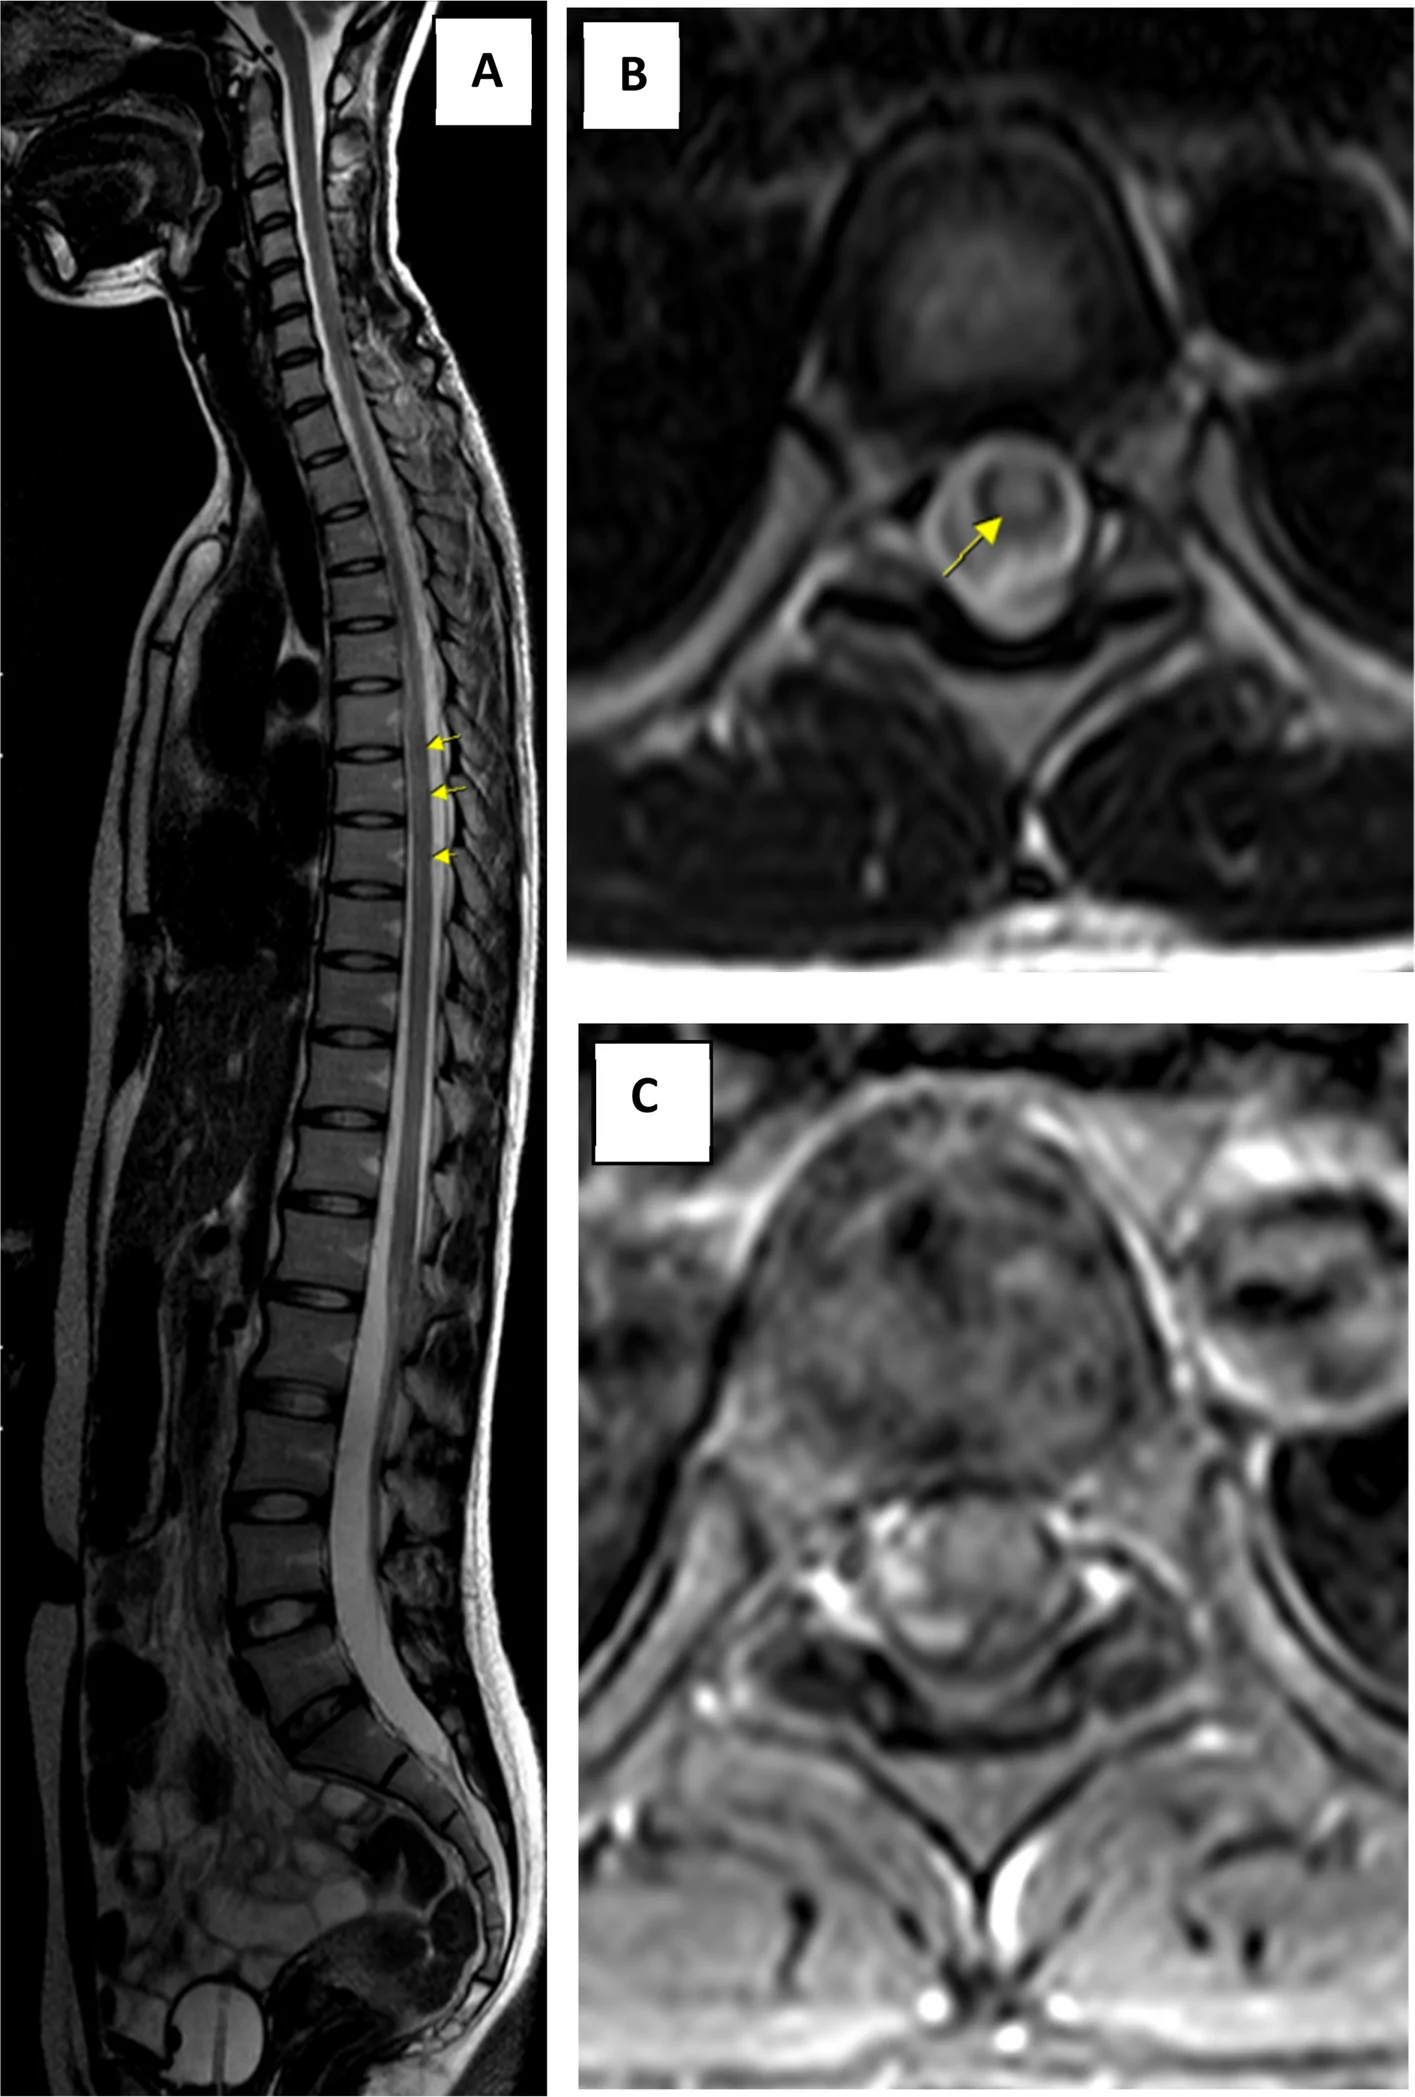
A Whole-spine sagittal T2-weighted imaging (T2WI) demonstrates a faint hyperintense lesion at the T6–T7 and T7–T8 levels (arrows), which is more clearly visualized on the corresponding axial image (B, arrow). No contrast enhancement is observed on post-contrast imaging (C)
